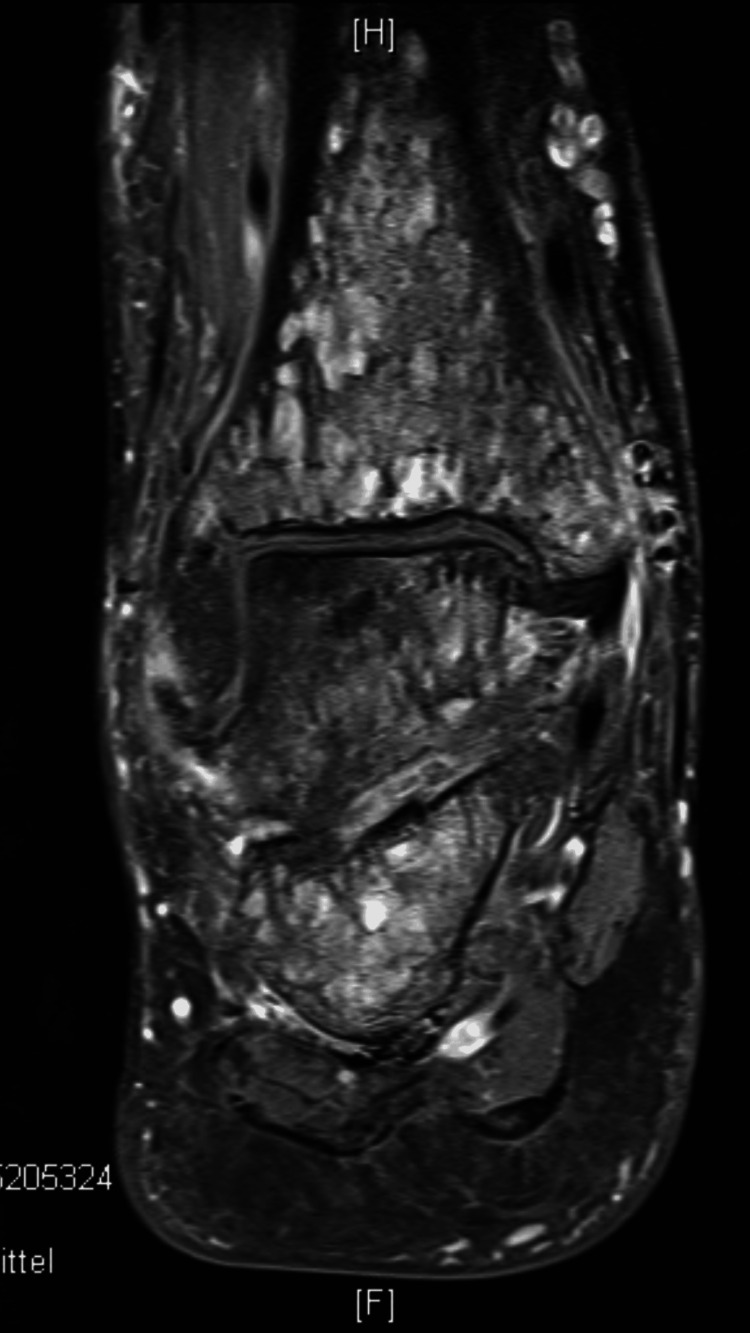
Coronal gadolinium-enhanced MRI demonstrated multiple lesions involving the tibia, talus, and calcaneus.
